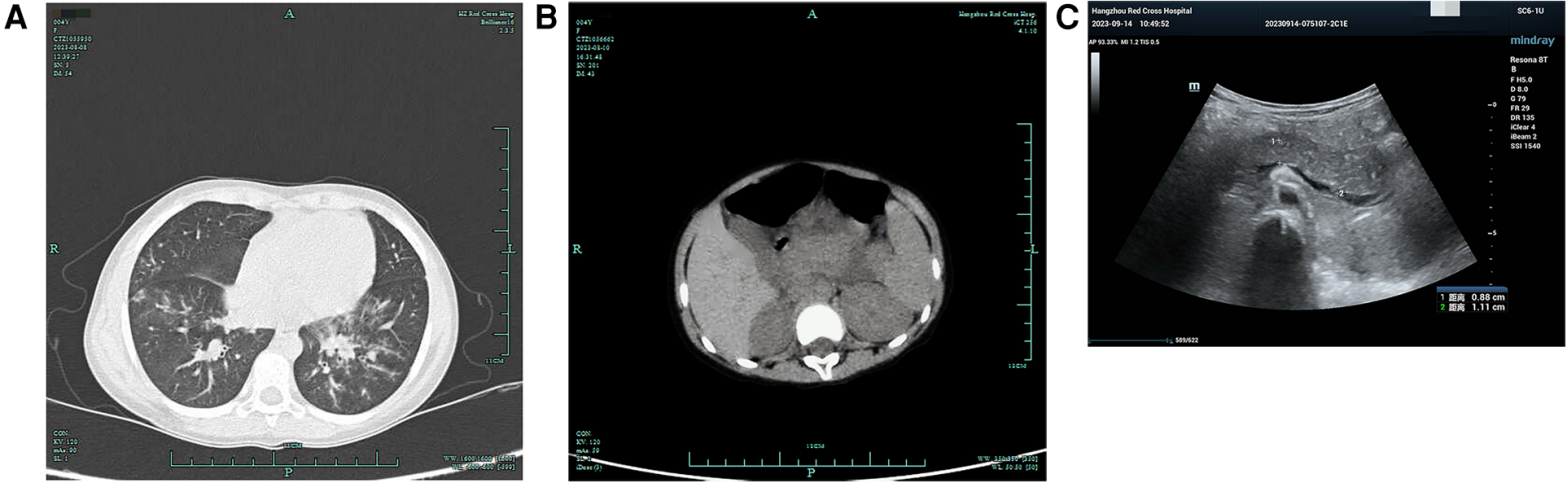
Lung and pancreas images. (A) Lung CT showed scattered patchy high-density blurred shadows in both lungs, without pleural effusion and thickened pleura. (B) Abdominal CT showed pancreatic enlargement with poor visualization, blurred surrounding spaces, and occasional fluid accumulation shadows. (C) Abdominal ultrasound showed the pancreatic head, body, and tail measured approximately 1.7, 1.4, and 1.3 cm in thickness, respectively. There was a significant fluid accumulation of approximately 1.0 cm in the intestinal gap. Fluid accumulation could be seen in the liver and kidney crypts, as well as the spleen and kidney crypts, with a maximum depth of approximately 0.7 cm.
